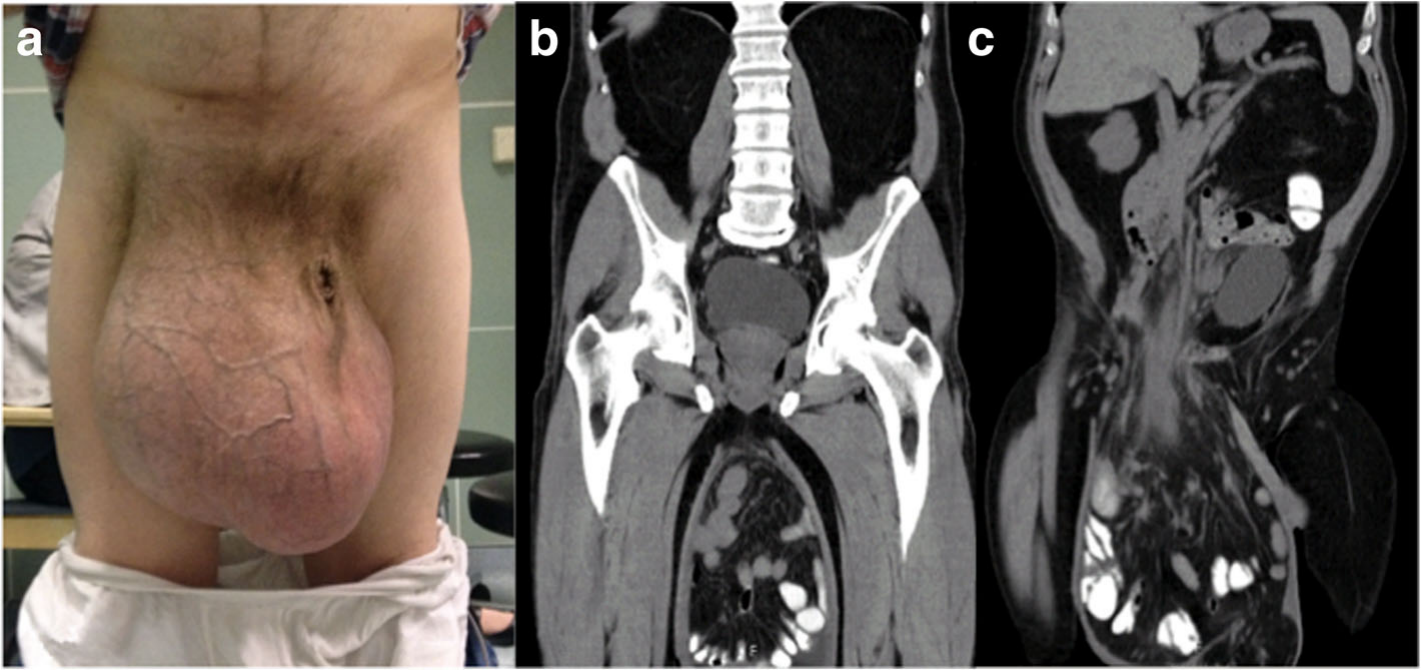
Frontal view of the giant inguinoscrotal hernia descending to the level of the knees (a). Computed tomography of the entity displaying subtotal evisceration of small bowel and ascending and transverse colon with intact vascularization (b/c)
